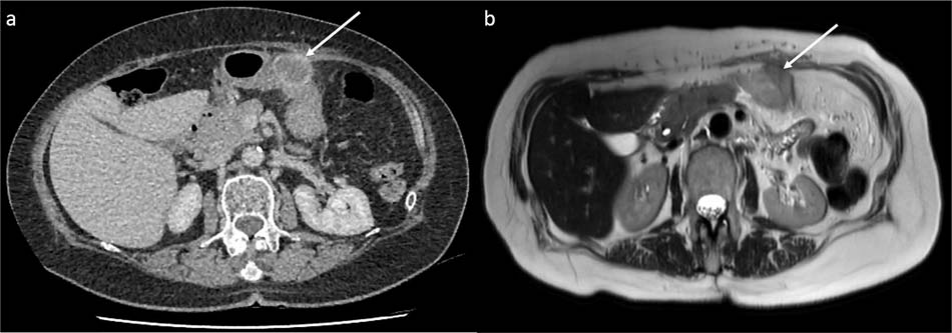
CT and MRI of the lesion (arrows). (a) CT showed a 32 mm cystic enhancing lesion between the anterior abdominal wall and mid greater curvature of the stomach. (b) The lesion appeared hyperintense on T2 imaging and predominantly isointense on T1 imaging, without significant internal debris.
